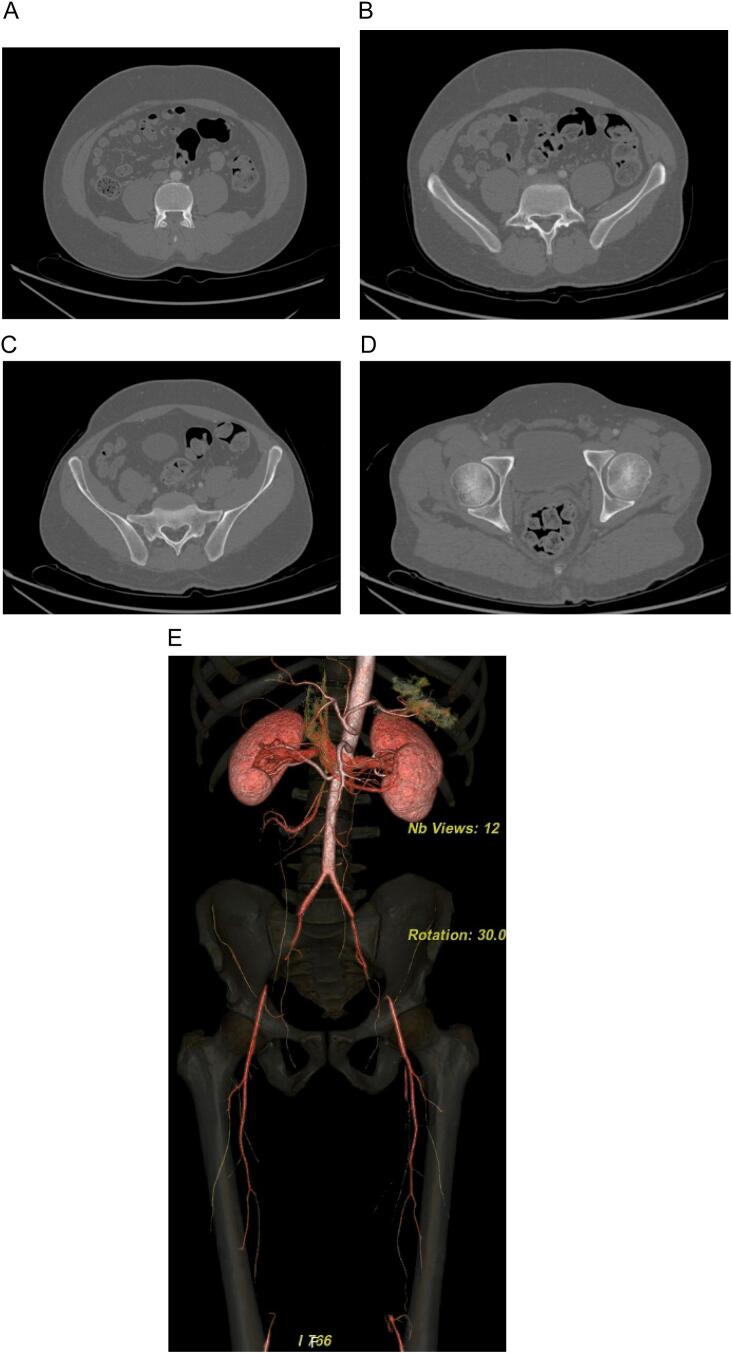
Figure 1: CT angiography of lower abdominal aorta and lower extremities. 1A: Patent distal aorta, just proximal to the bifurcation. 1B: Patent bilateral common iliac arteries. 1C: Occluded bilateral external iliac arteries, and patent bilateral hypogastric arteries. 1D: Reconstruction of flow in both common femoral arteries through collaterals. 1E: 3D reconstruction of his lower limb angiography showing occluded external iliac arteries and proximal two thirds of both superficial femoral arteries.
